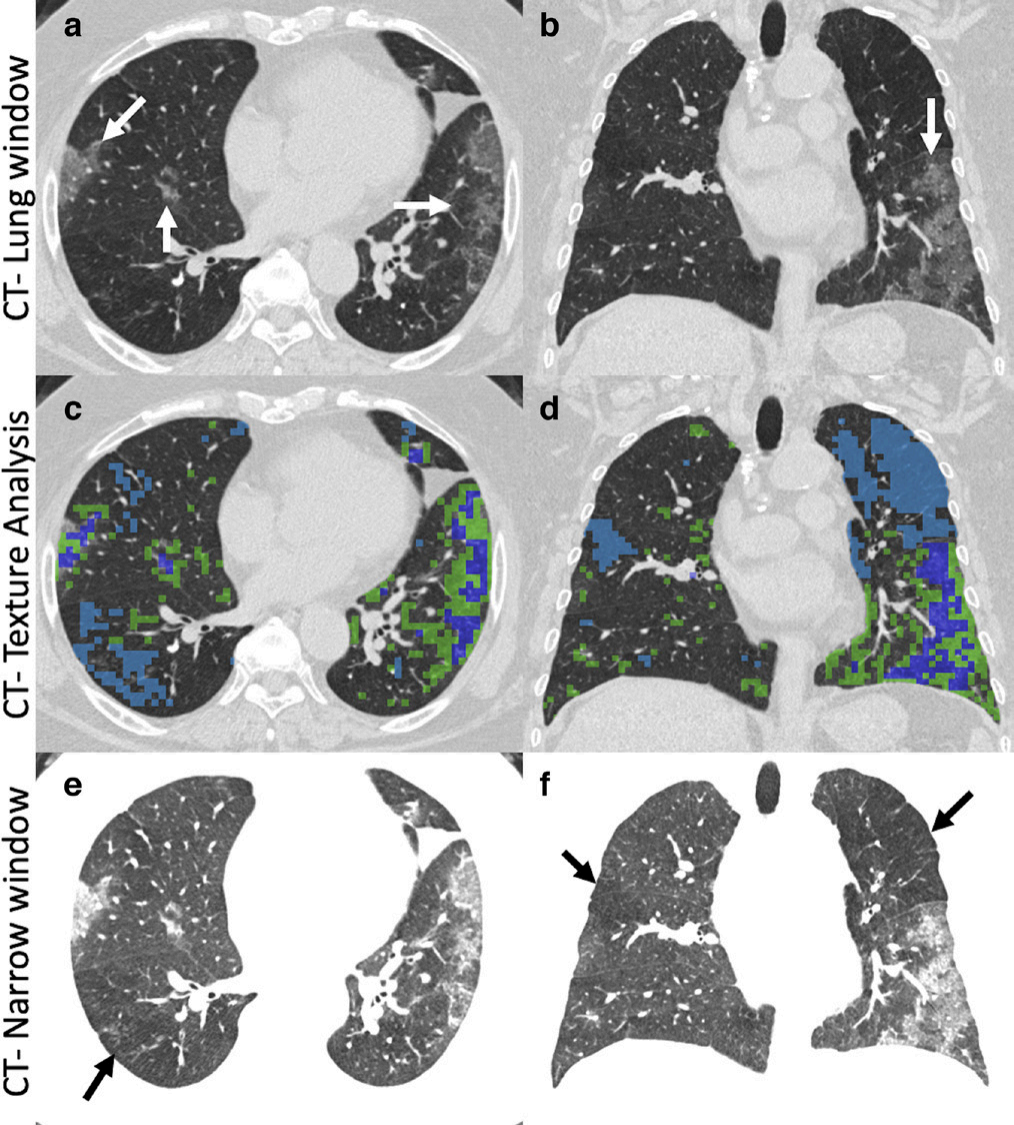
Conventional CT lung window images (A, B) in a patient with COVID-19 pneumonia showing typical or common peripheral predominant disease. 3D AMFM texture analysis (C, D) characterized the diseased lung as GGO(green color) and reticular interstitial thickening superimposed on GGO (dark blue). Additionally, areas of ‘emphysema-like’ texture (light blue) were identified. While no emphysema was seen on lung window images, the high contrast narrow window settings (E, F) show that these areas are hypodense (black arrows) presumably related to microthrombi or regional hypoxic vasoconstriction. AMFM, Adaptive Multiple Feature Method; GGO, ground-glass opacity
